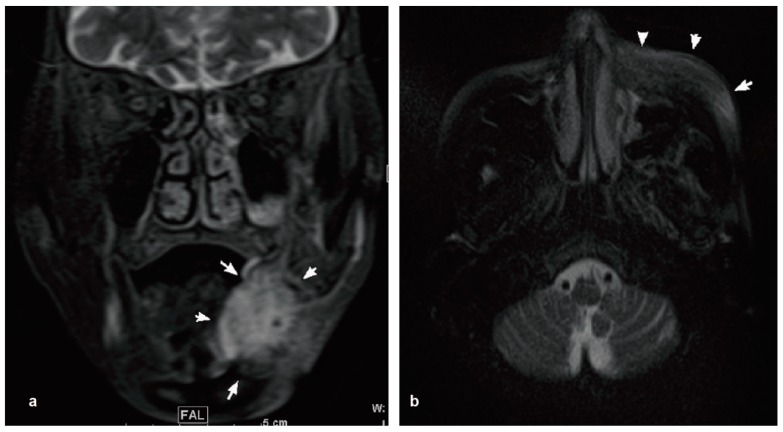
MRI indicated the swelling of the left submandibular tissue (arrowhead). (a) Coronal scan contrast T2; (b) Axial scan contrast T2.
